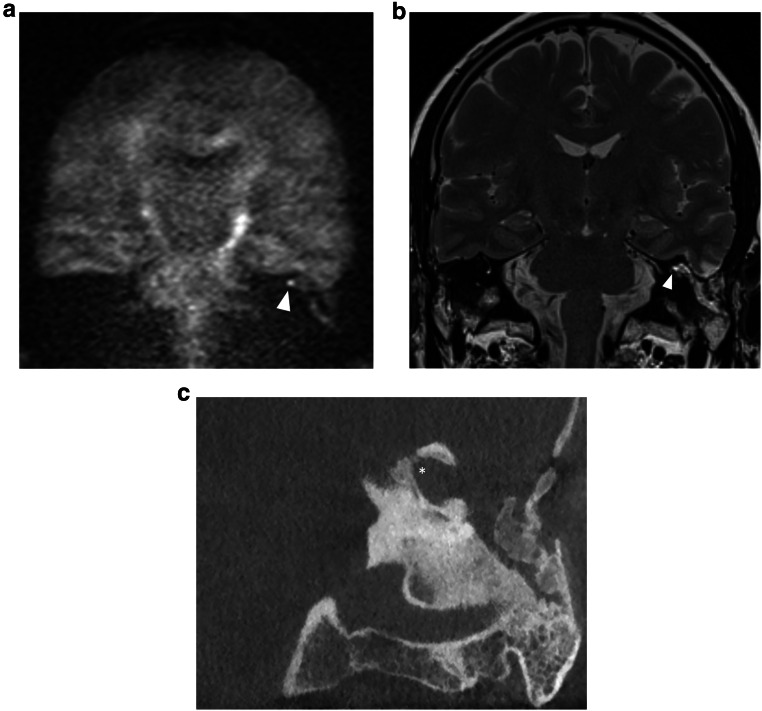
Same case as in Fig. 6, three years after initial diagnosis and surgery. MRI of the posterior fossa shows a focus of restricted diffusion (a) with corresponding high signal intensity on the T2-WI (b), in keeping with residual CC (arrowheads). c CBCT of the temporal bone shows soft tissue filling the mastoid cavity but the residual disease is not discernable (asterisk)
